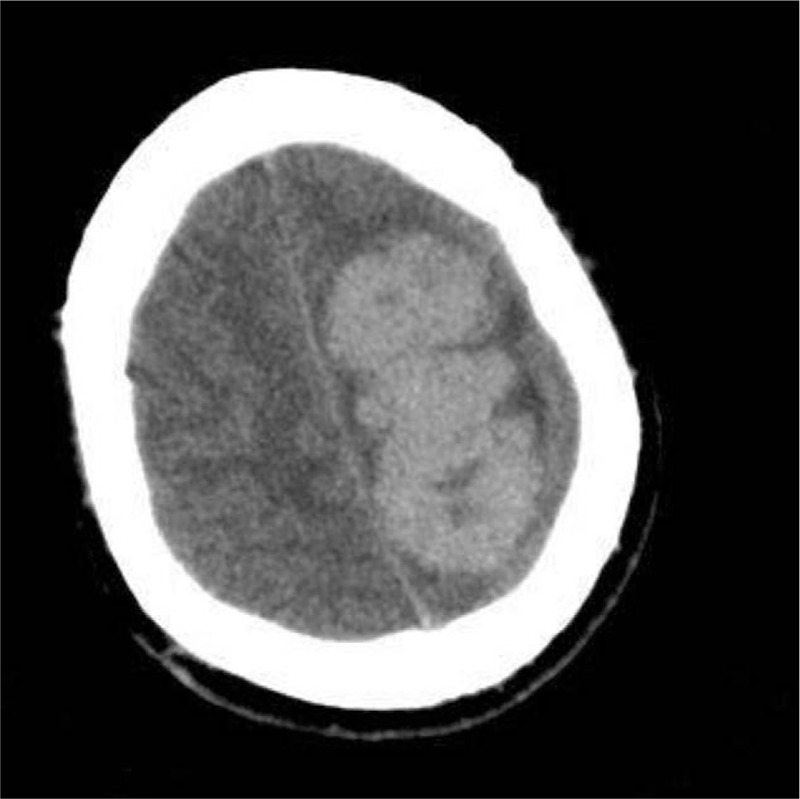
CT scans showed a high-density lesion in the left frontoparietal lobe. CT = computed tomography.
